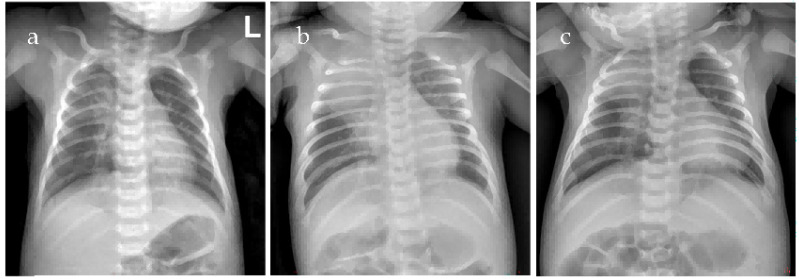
Chest radiograph images of Case 1 (a–c). Image (a) obtained at admission demonstrates mild patchy opacity, while both (b) obtained prior to the first bronchoscopy and (c) obtained prior to the second bronchoscopy reveal bilateral faint patchy opacities and consolidation, predominantly in the right upper lung zone.
